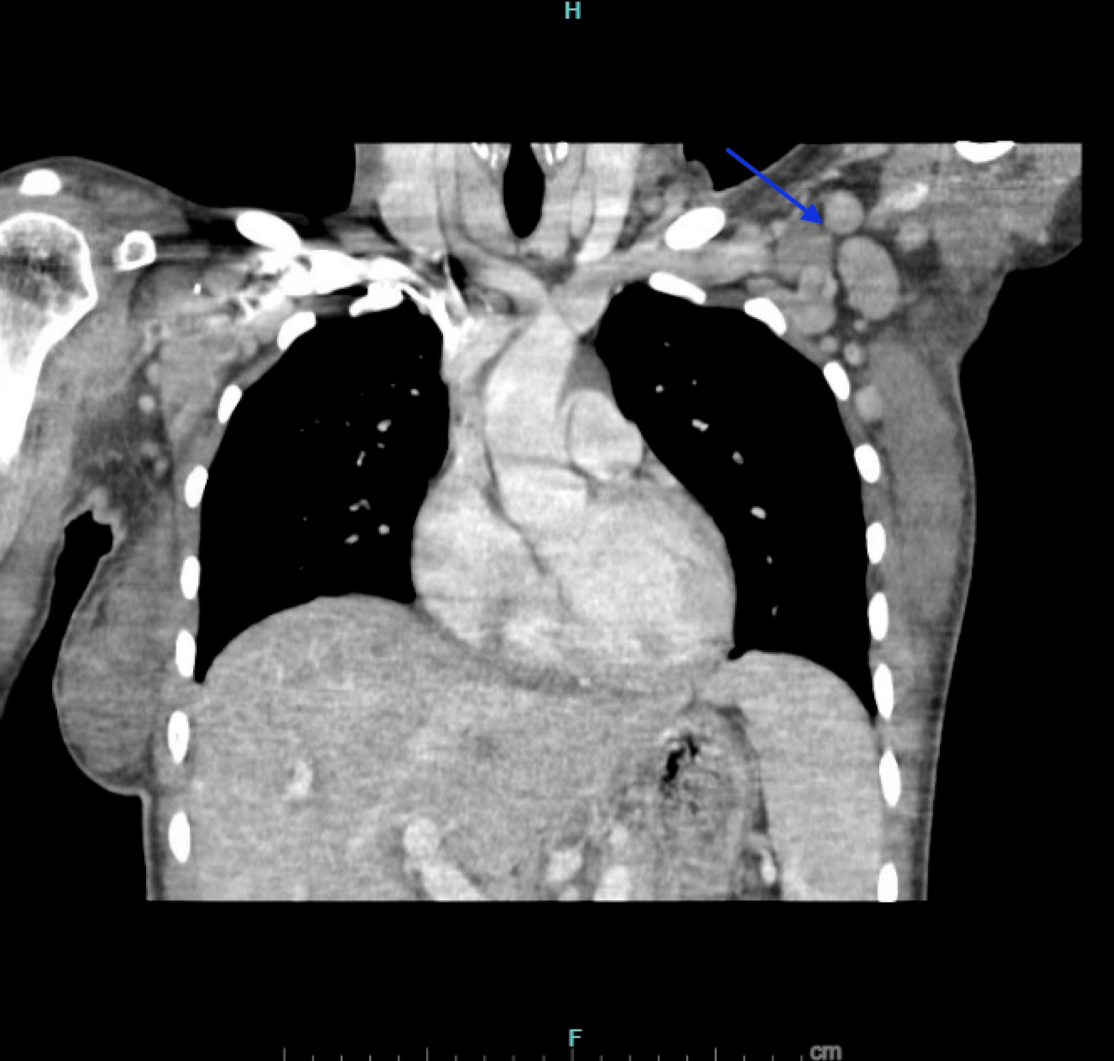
CT Chest with Intravenous Contrast in the Coronal Plane The blue arrow shows lymphadenopathy in the left axilla. CT: Computed Tomography
